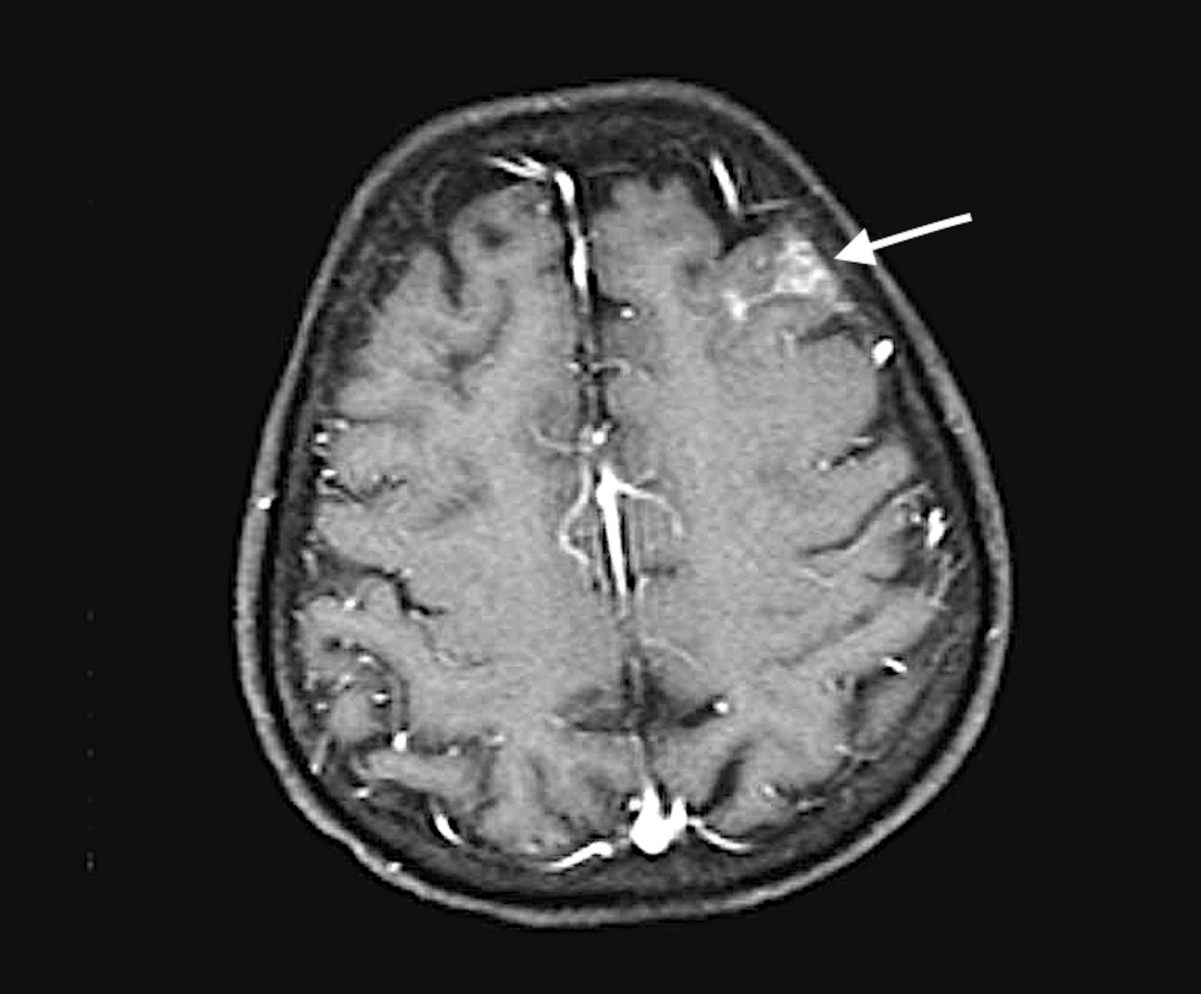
Magnetic resonance imaging (MRI) of brain depicting left frontal lobe subarachnoid hemorrhage (arrow).
